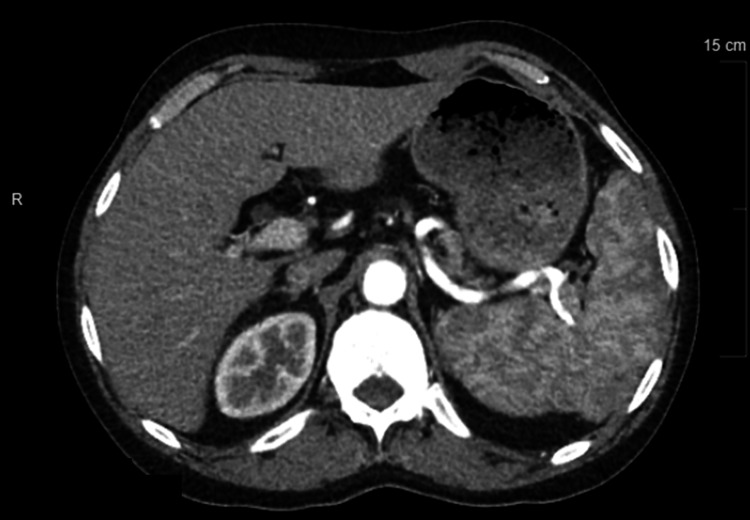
Preoperative axial CT with contrast showing heterogeneous uptake in the enlarged spleen.
